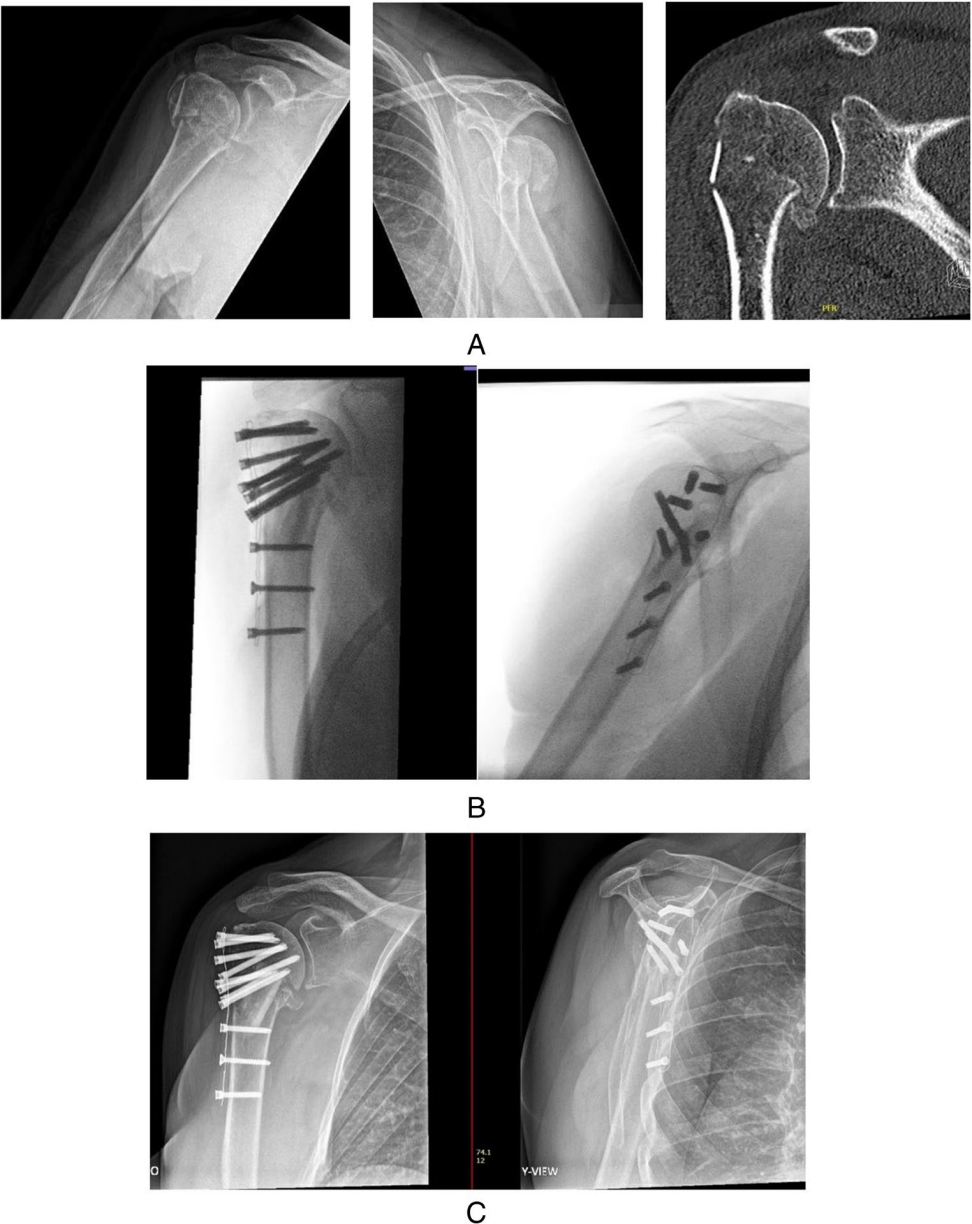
A 62 year old female with a 3-part PHF. A Fracture radiographic imaging and CT imaging, B Fluoroscopy image during surgical reconstruction, C Progressive consolidation at 9 months follow-up
